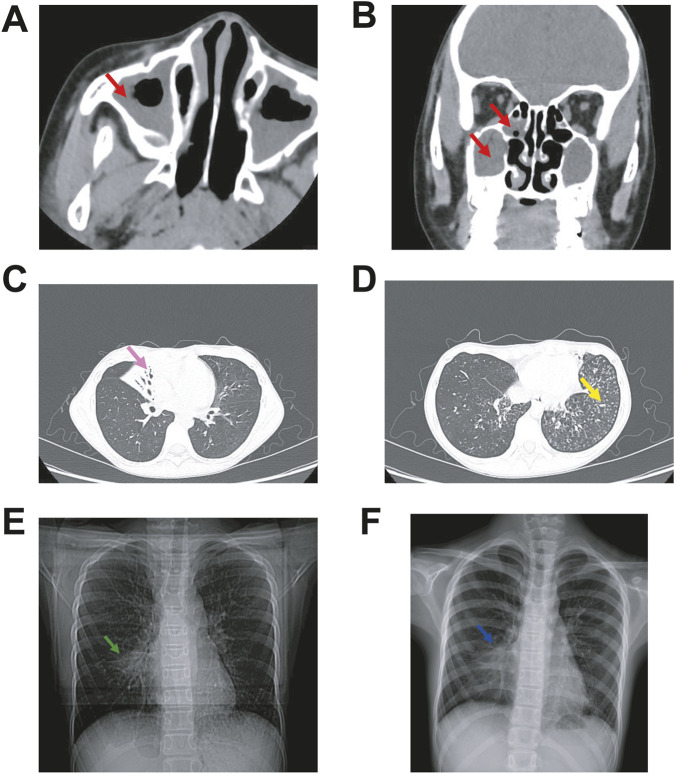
Paranasal sinus and chest computed tomography results. (A) and (B) Paranasal sinus CT results showed paranasal sinusitis, right inferior turbinate hypertrophy, and soft tissue thickening in the nasopharynx. Red arrow in (A) indicated mucosal thickening and increased density in the frontal sinus; Red arrow in (B) denoted mucosal thickening and increased density in the ethmoid and maxillary sinuses (C,D) Chest CT results demonstrated bilateral pulmonary infectious lesions with partial consolidation, focal bronchial dilation. Pink arrow in (C) presented atelectasis with consolidation in the right middle lobe, with visible bronchial dilation; Yellow arrow in (D) showed a “tree-in-bud” pattern in the left lung. (E) Coronal scout view of the chest CT showed patchy opacities with ill-defined margins in the right lower lung field adjacent to the mediastinum (indicated by the green arrow) (F) Chest radiography presented increased and blurred bronchovascular markings bilaterally in the proband. Multiple patchy, ill-defined opacities with diffuse distribution were observed in the left lower lung field. The blue arrow denoted the pathological features in the right lower lung field adjacent to the mediastinum were consistent with the findings from the coronal scout CT image.
